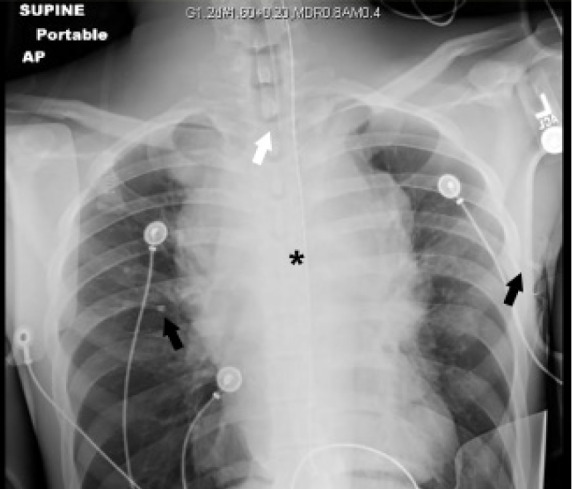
Supine anteroposterior chest radiograph. Asterisk identifies a centrally located opacity that obscures the normal mediastinal anatomy including the trachea, pulmonary vasculature and aortic contour. Black arrows denote bilateral angiocatheters used for decompression of the chest. White arrow identifies the tip of the endotracheal tube at the level of the clavicles. The lungs appear unremarkable.
